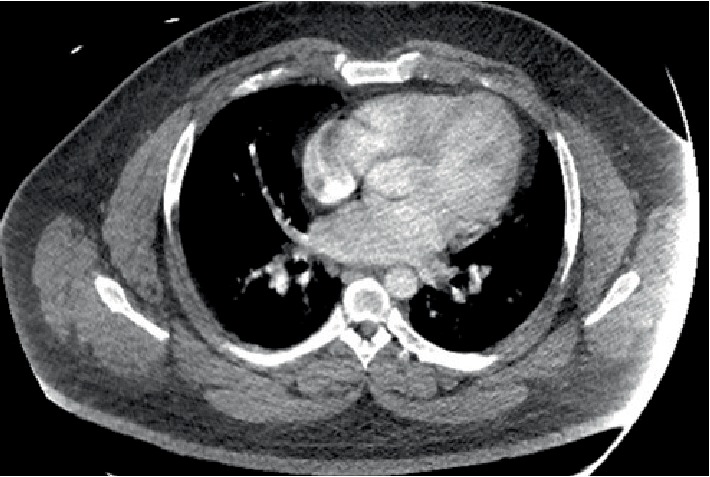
CT angiogram Chest with intravenous contrast shows small to moderate left lower lobe pulmonary emboli. A 2 cm pleural-based right lower lobe nodular density can also be seen. It should be noted that evaluation of the pulmonary arteries is suboptimal secondary to patient body habitus and under opacification of the pulmonary vessels.
